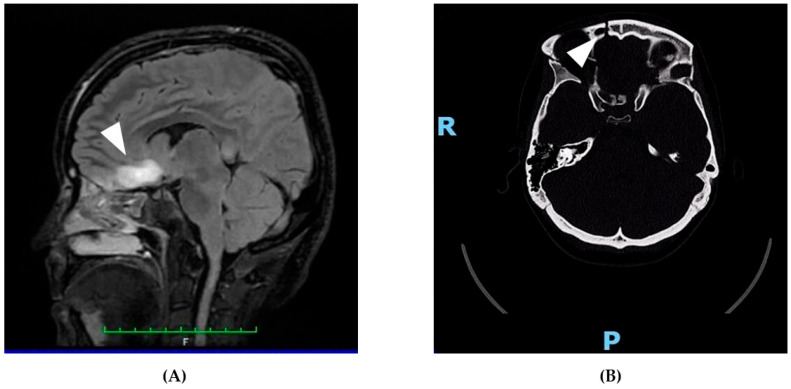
Representative neuroimaging findings following severe traumatic brain injury. (A) Sagittal FLAIR MRI showing a hyperintense lesion in the pericallosal region and pituitary stalk, consistent with diffuse axonal injury and cytotoxic edema. These findings support the neuroanatomical basis for the patient’s cognitive, executive, and neuroendocrine symptoms. (B) Axial non-contrast CT scan revealing a right orbitozygomatic complex fracture with disruption of the orbital wall, consistent with the patient’s initial ocular motility impairment and diplopia. This image demonstrates the extent of craniofacial trauma sustained at the time of injury. The MRI images are original and were obtained as part of the patient’s routine clinical assessment. All images were fully anonymized, and written informed consent for publication was obtained. No copyright permission is required.
